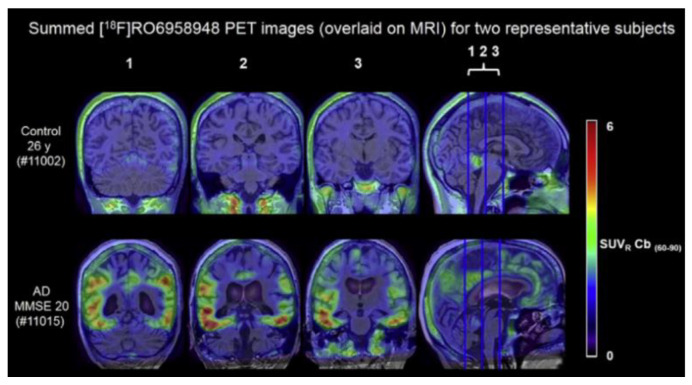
(18F)RO-948 PET images overlaid on MRI on a healthy control (upper row) and an AD patient (bottom row). The increased uptake of (18F)RO-948 in the AD patient is associated with the binding to the hyperphosphorylated tau protein. [80]. Images are reprinted with permission of the publisher [80].
